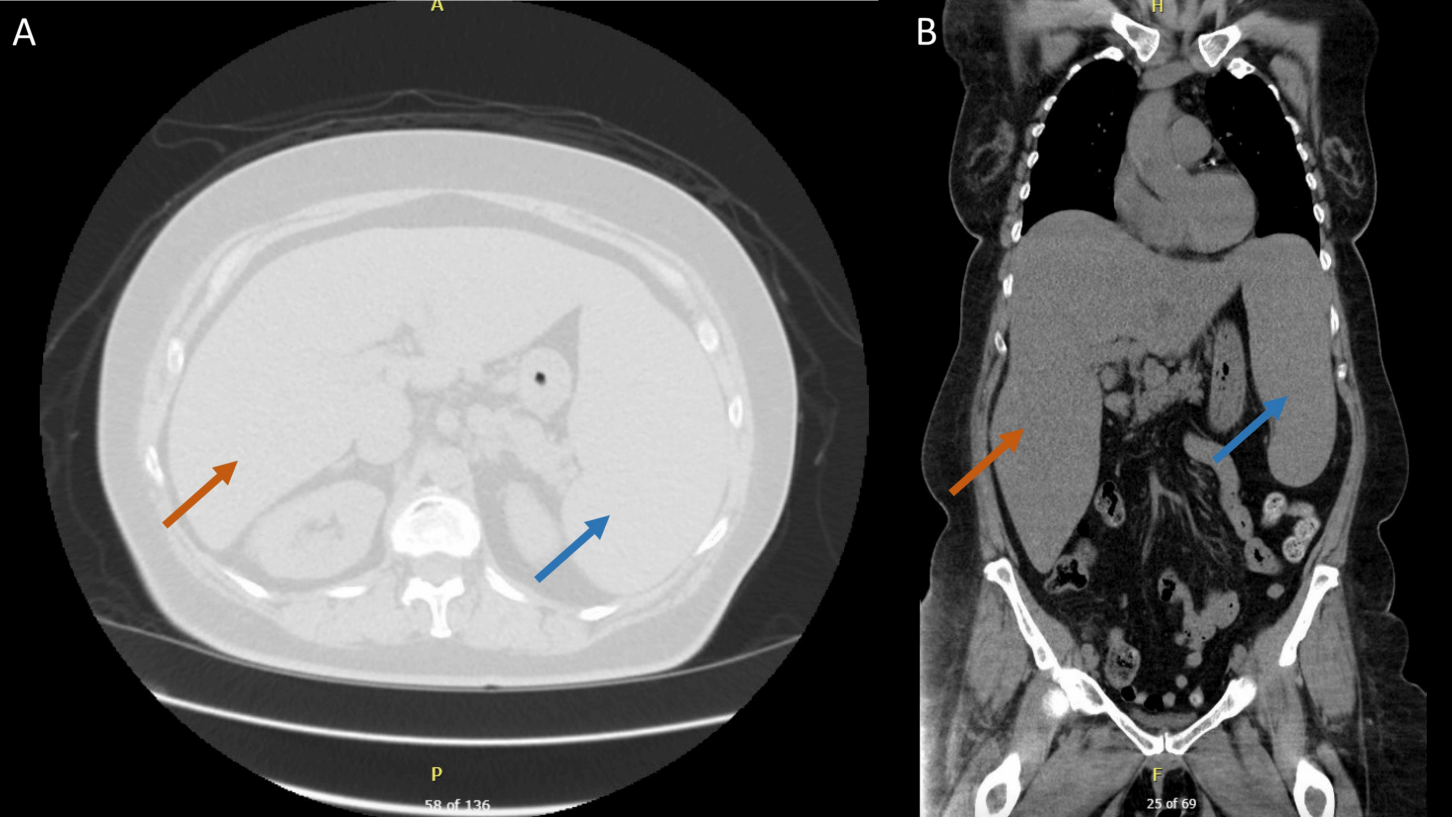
CT abdomen showing hepatomegaly (red arrow) and splenomegaly (blue arrow) A: axial section; B: coronal section
